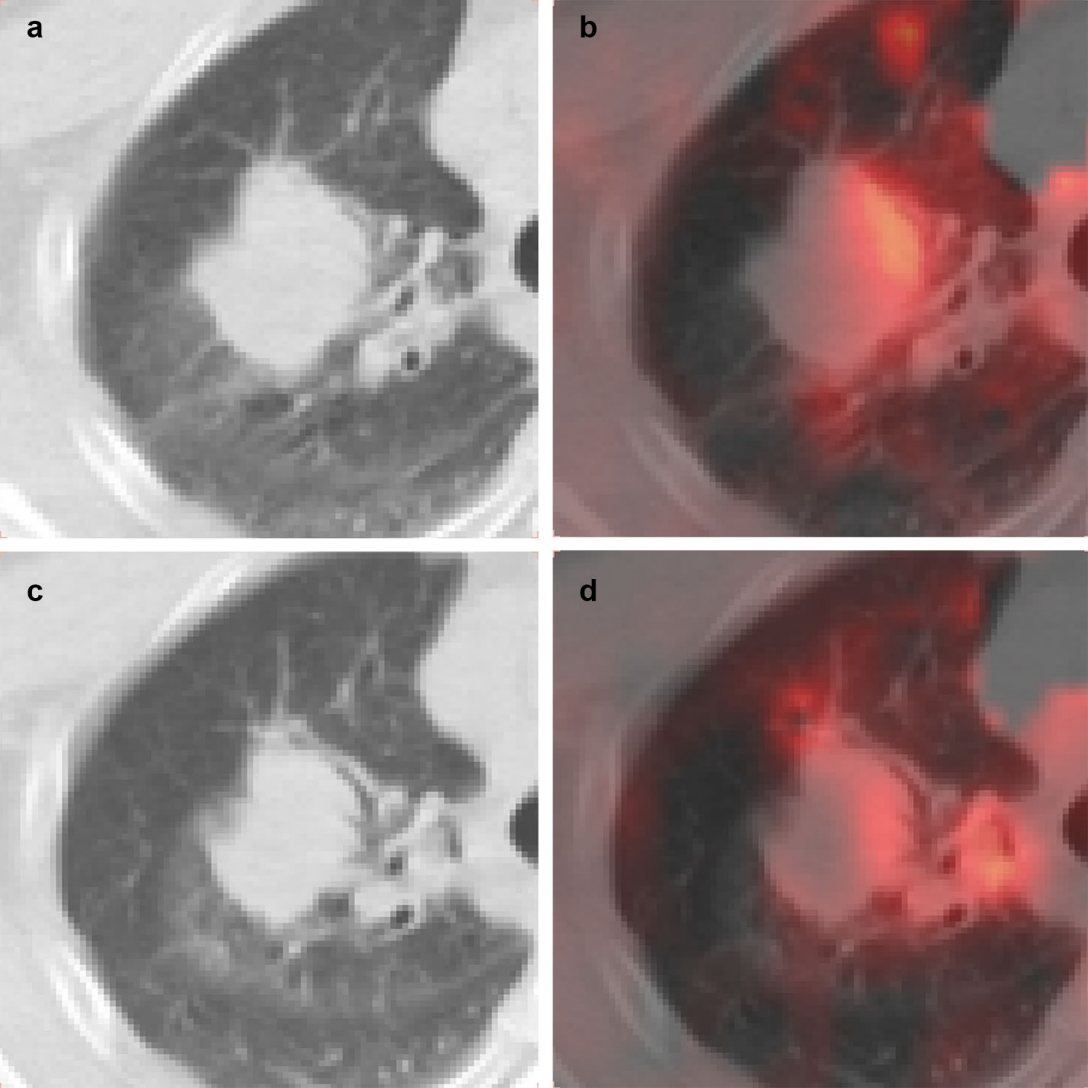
^15^O-water PET image of a 62-year-old man with adenocarcinoma treated with bevacizumab (patient no.1). (a,b) CT and fusion image of CT and parametric image of blood flow at baseline. (c,d) CT and fusion image after chemotherapy. The CT showed a 4.4 cm large mass in the right lung. Tumor blood flow was 0.371 mL/mL/min before treatment and slightly decreased to 0.334 mL/mL/min after chemotherapy (blood flow ratio: 0.90). He had no tumor progression for 1 year and 2 months.
